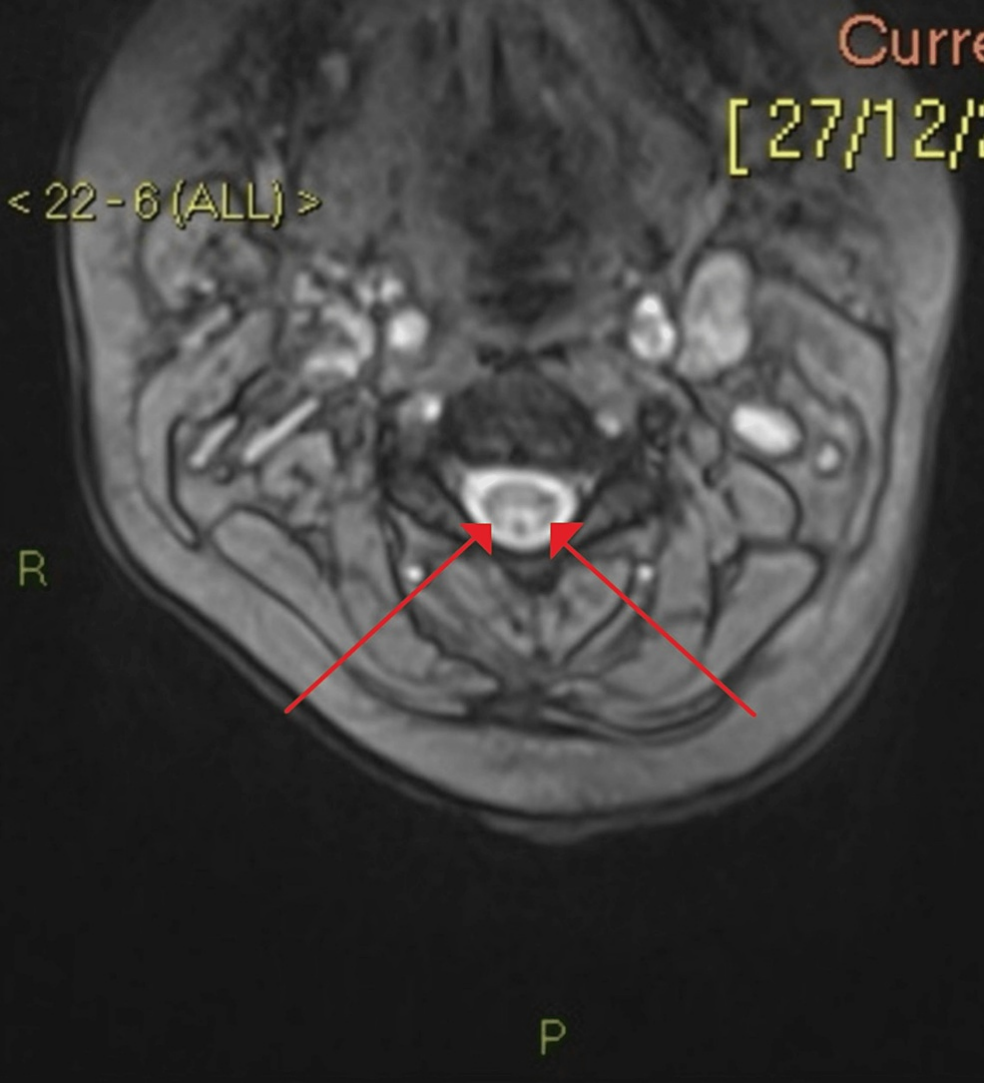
MRI spine demonstrating symmetrical high signalling in the dorsal column/posterior spinal cord from C2-C6
